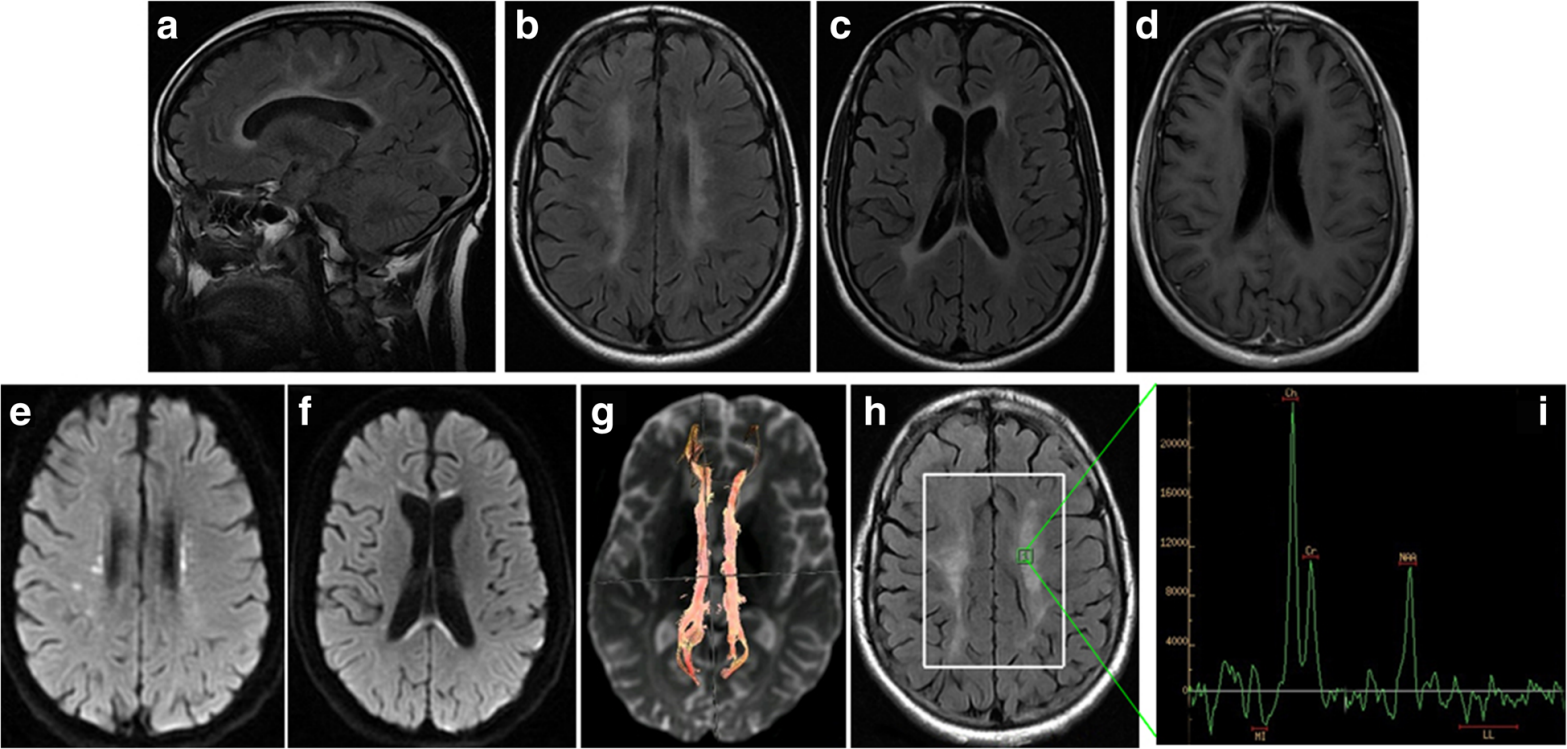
Brain MRI, DWI, DTI, and MRS images. T2/Flair showed multifocal periventricular white matter lesions (a b and c), without enhancement (d). DWI shows high-signal intensities in periventricular white matters and corpus callosum (e f). DTI shows decreased numbers of corpus callosum fibers, while subcortical arcuate fibers are spared (g). MRS shows increased Cho levels, while NAA levels are decreased in the white matter lesions (h i)
